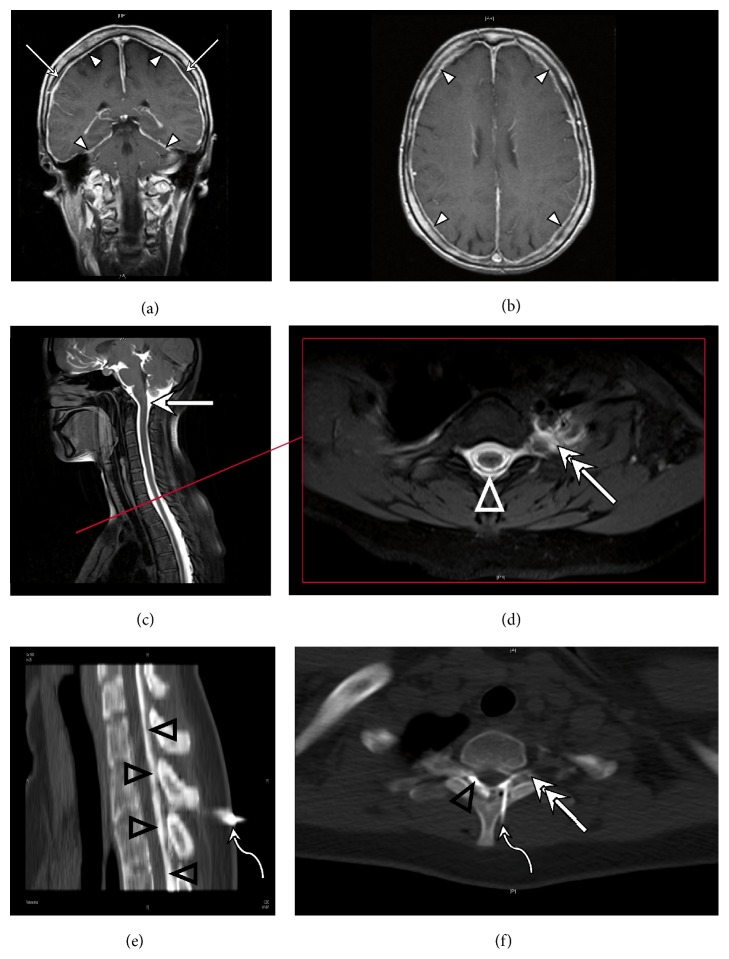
Imaging in a patient with symptomatic CSF leakage. (a + b) MRI with intravenous contrast media displays enhancement of the meninges over the convexity and tentorium (arrow heads). A fluid collection separates the periosteal and the inner layer of the enhancing dura mater (arrows). (c + d) MRI with intrathecally applied gadolinium contrast agent. (c) Distribution of contrast agent in the subarachnoid space (solid arrow). (d) MRI depicts transforaminal contrast leakage as the diagnosis of meningeal tears in the left T2 nerve root (double arrow) with epidural distribution of contrast agent in the epidural space (triangle). (e + f) CT-guided blood patch. (e) Tuohy needle in the epidural space of T1/2 (curved arrows) used to inject a mixture of blood and iodine contrast agent into the epidural space (black triangles). (f) Epidural blood patch by means of a Tuohy needle (curved arrow) with epidural (triangle) and transforaminal (double arrow) distribution of the blood at the level of the T2 nerve root.
